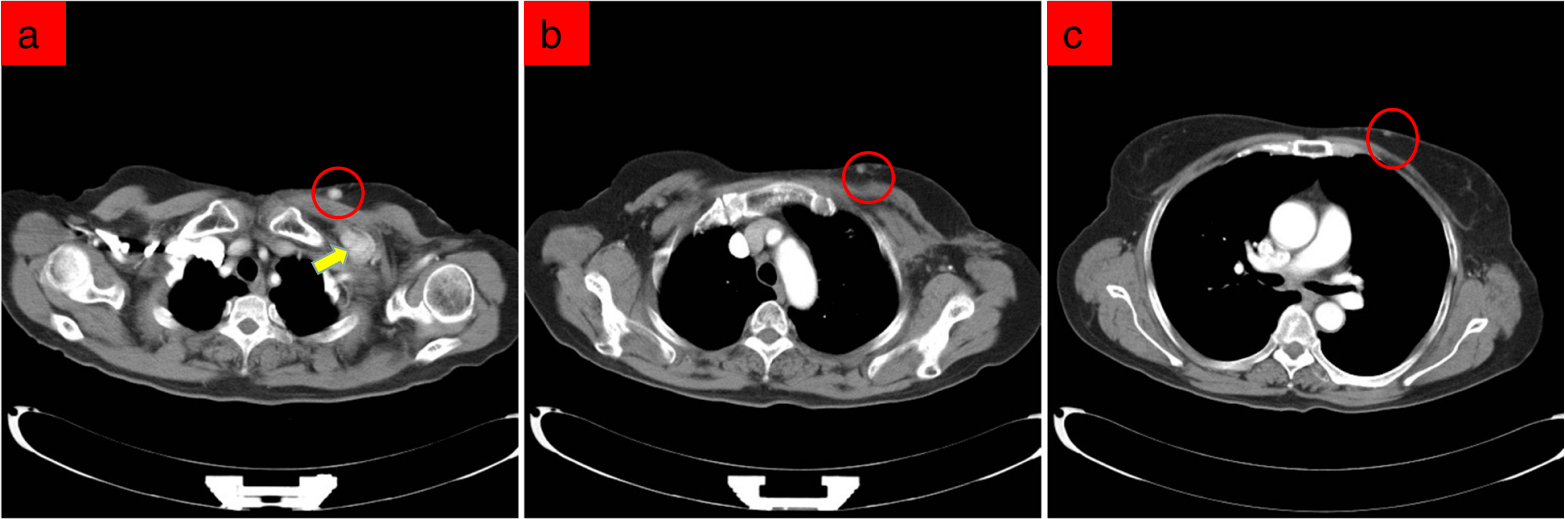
CT scan showing the shadow of the horizontal cutaneous nodules (red circle) in the left subclavian region (a) and the second (b) and third (c) intercostal spaces of the left anterior chest wall. The largest one is in the left subclavian area, which measured approximately 3 × 3 cm (yellow arrow); the density was uniform, the boundary was clear, the enhancement scan was non-uniform and significantly enhanced, and lymph node metastasis was considered. A B
